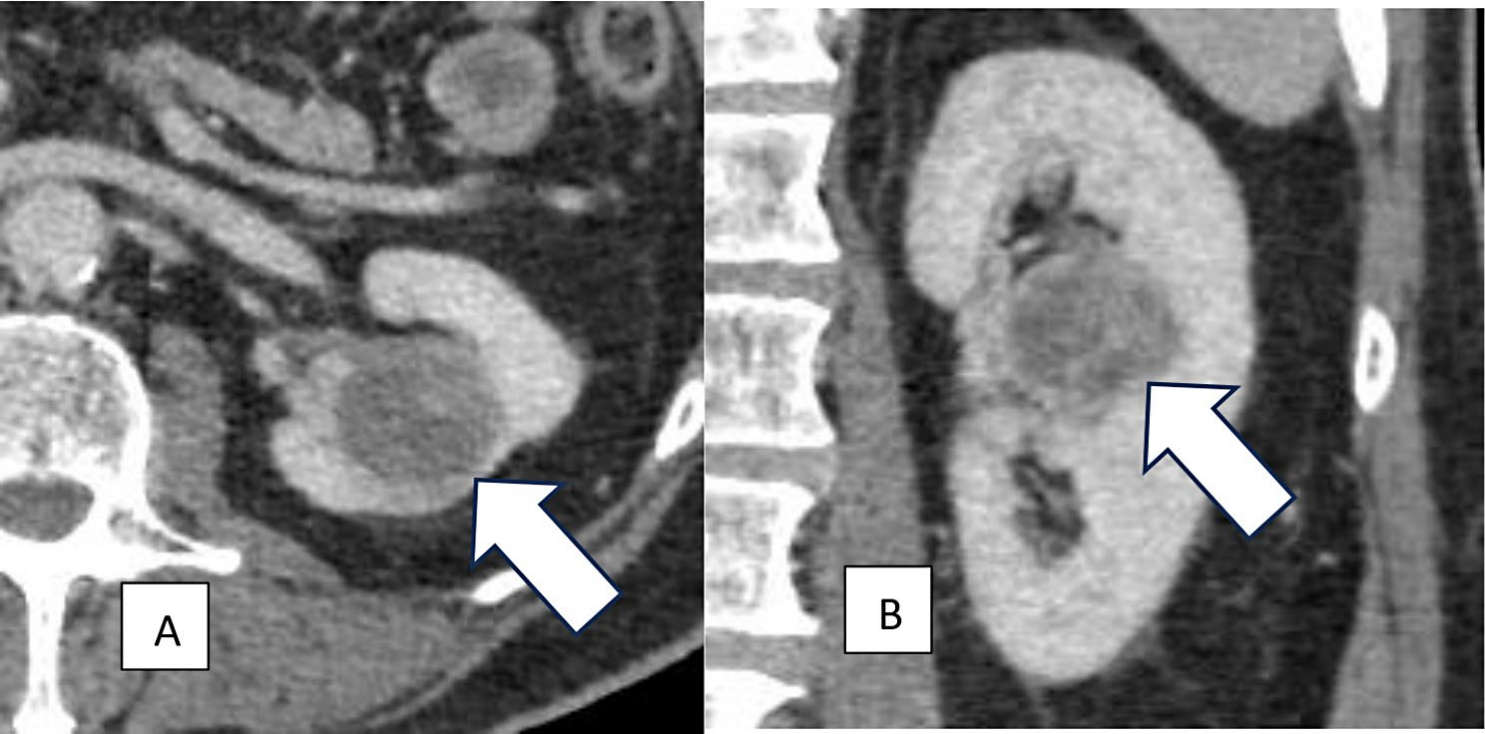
(A): Contrast-enhanced axial (A) and coronal CT images (B) of the abdomen at the level of the left kidney show an endophytic, circumscribed renal mass. While renal sinus invasion can be subtle and often challenging to detect on CT scan (such as in this case), pathology results are essential for accurate diagnosis and staging. Pathology results in that case were positive for clear cell RCC with renal sinus involvement, representing pT3a disease
